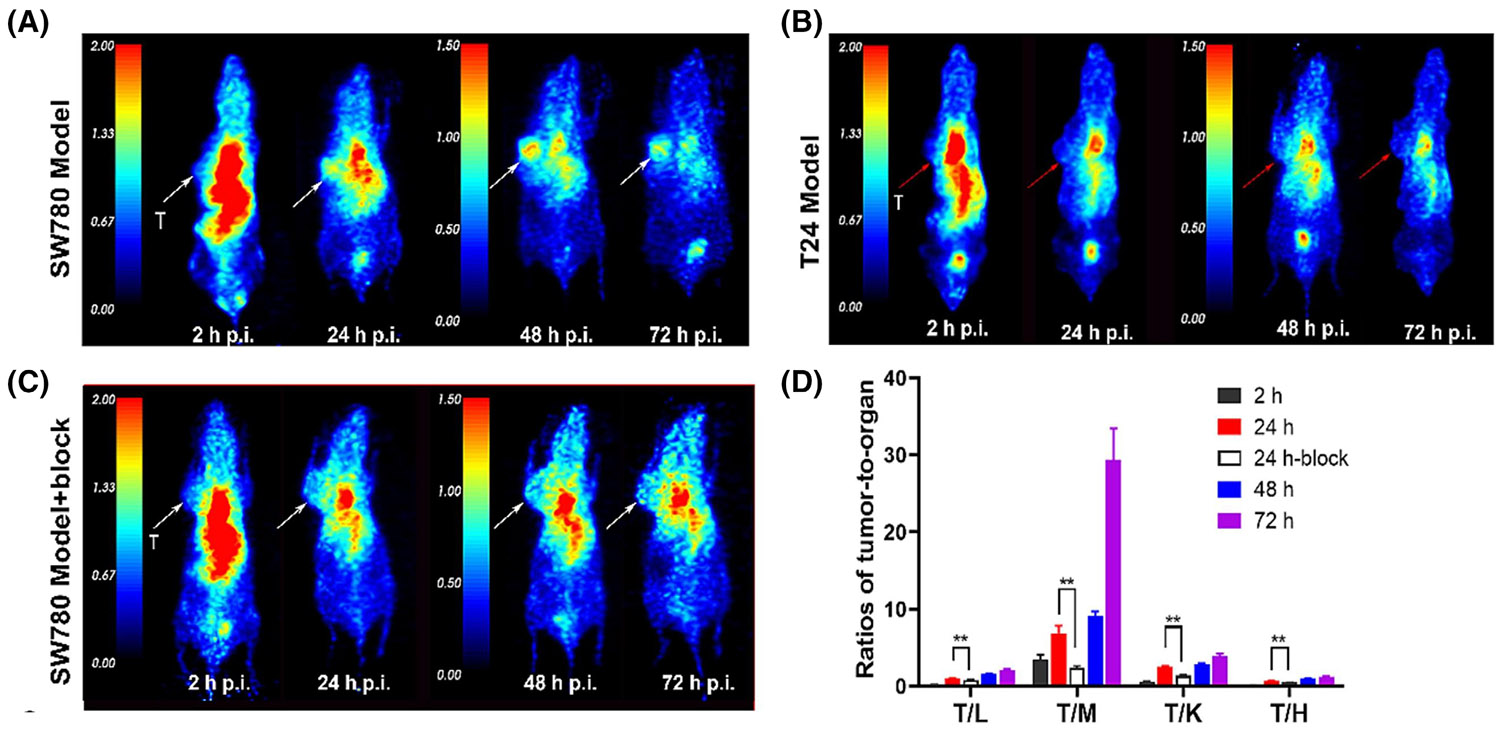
^124^I-EV PET/CT imaging enables visualize UC. PET images of ^124^I-EV in the SW780 xenograft model without blocking (A) and with blocking (B) and in the T24 xenograft model (C). The SW780 and T24 tumors are denoted by white and red arrows, respectively. (D) Ratios of tumor-to-organ according to ROIs. Reproduced with permission.^60^ Copyright 2024, Elsevier. PET, positron-emission tomography; UC, urothelial carcinoma; ROI, region of interest.
